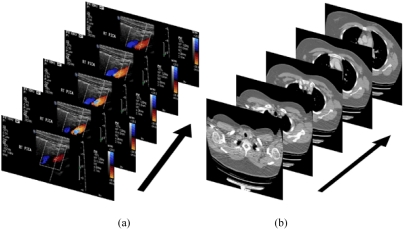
(a) Ultrasound clips use the 3rd dimension to represent time; (b) CT volumetric images use the 3rd dimension to represent depth.
